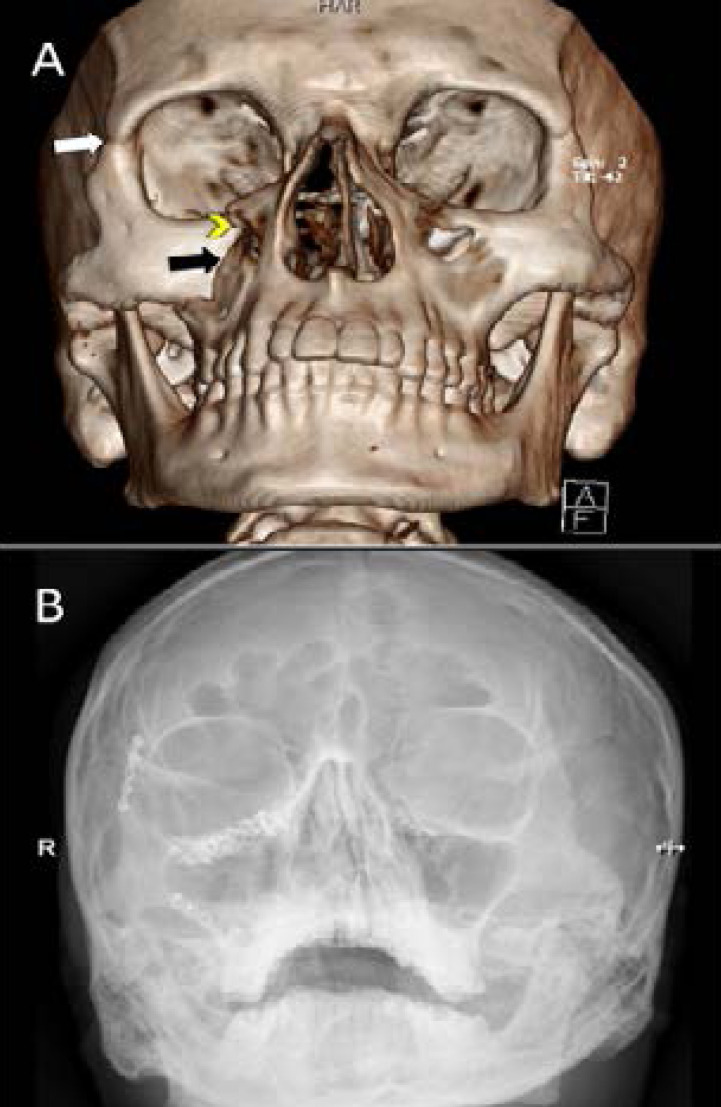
A 31-year-old camel caregiver was kicked by a camel into the face. A three-dimensional (3D) CT scan (A) demonstrates a depressed fracture of the right zygomatic bone (black arrow), fracture of the right lateral orbital wall (white arrow), and orbital floor (yellow arrowhead). Postoperative plain radiograph (B) showing reconstruction of facial fractures with open reduction and internal fixation.
